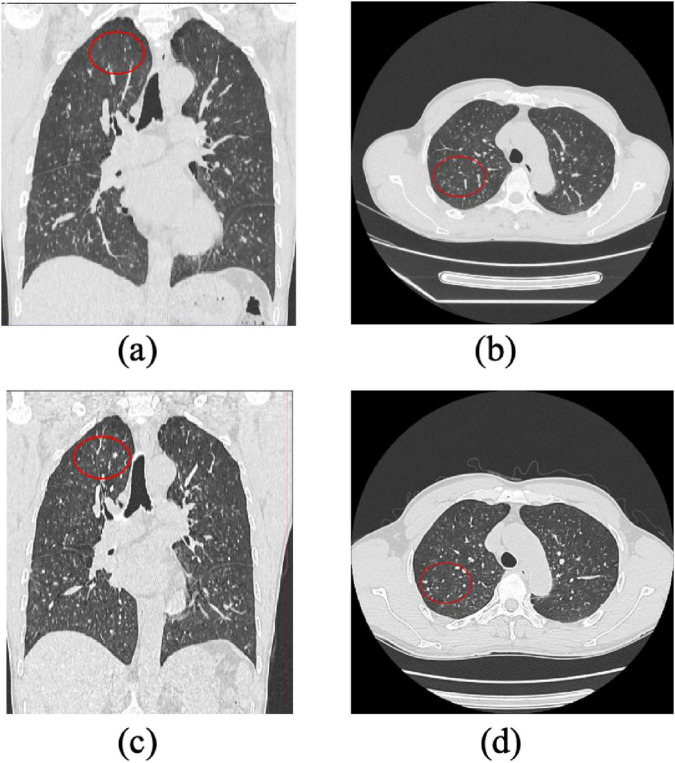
Progression of Pulmonary Lesions in a Stage I Pneumoconiosis Patient: A 1-Year Follow-Up CT Study. (a) and (b): Initial CT scans show multiple small high-density nodular opacities and linear fibrotic streaks distributed in the bilateral upper and middle lung fields, primarily affecting the upper lobe segments and lower lobe dorsal segment. (c) and (d): Follow-up CT images taken 1 year later reveal an increased number of nodular and fibrotic lesions in the same lung regions, indicating progression of fibrosis. The findings suggest disease advancement over time.
